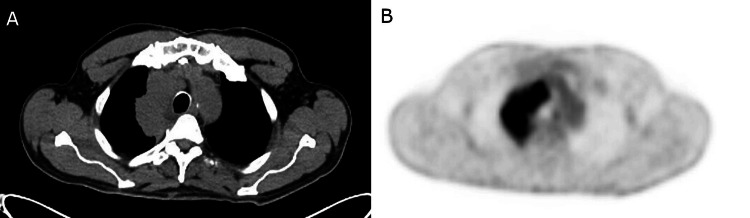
Radiology findings. (A) Chest CT: Right suprahilar/mediastinal conglomerate mass measuring approximately 8.1 x 4.9 x 9 cm. (B) PET scan: Large right hypermetabolic paramediastinal/hilar pulmonary mass concerning for malignancy.
